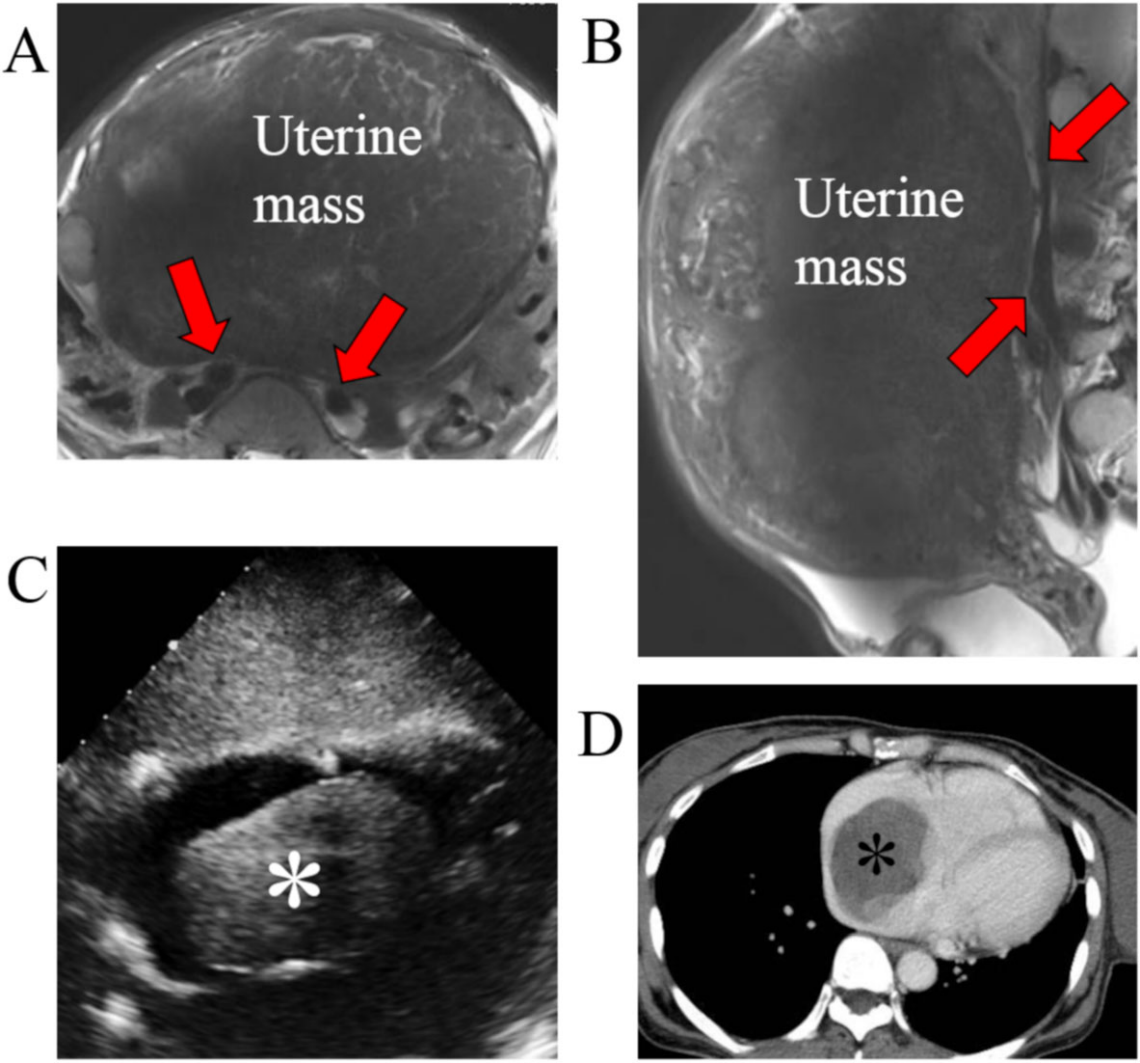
Clinical imaging findings: a and b T2-weighted MRI revealed a 27-cm mass in the uterus. No tumor was detected in the iliac vein (A, red arrows) or in the inferior vena cava (B, red arrows). c Echocardiography imaging revealed a 78 × 47-mm mass (asterisk) in the right atrium. d Enhanced computed tomography imaging confirmed a 75-mm mass (asterisk) in the right atrium
